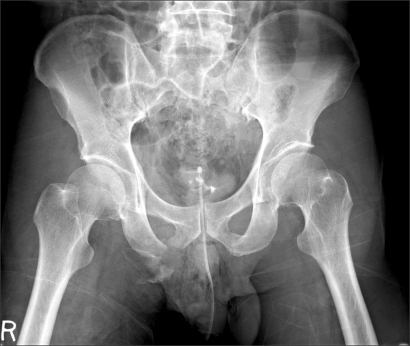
Radiograph showed concentric reduction of both hips
